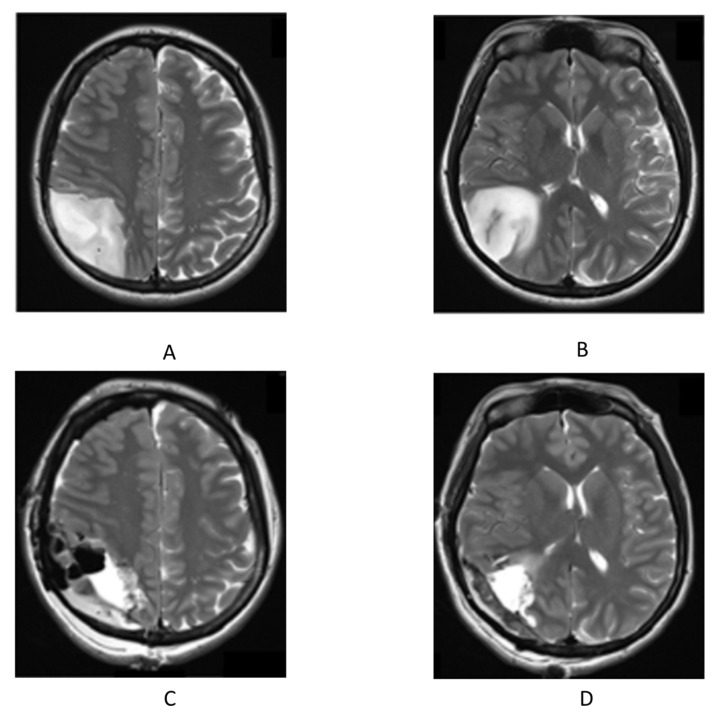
T2-weighted image of head magnetic resonance imaging. (A,B) before surgery; (C,D) after surgery.
